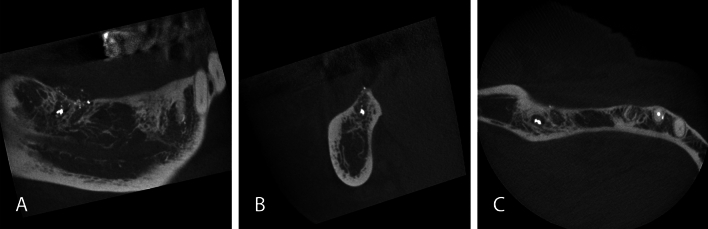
CBCT scan of a 71-year-old female patient with a small FOV (6 × 4 cm) for implant pre-treatment examination in free-end posterior right lower jaw. In the lower right wisdom tooth position a radiopaque concrement of dental filling material with circumferential radiolucency is visible. Furthermore, multiple small radiopaque concrements are recognizable in the residual socket and adjacent to the bone in the soft tissue (fragmented dental material in bone/in soft tissues). In the axial plane dish-like root remnants are identifiable in second premolar site, where implant placement was planned. Sagittal (A), coronal (B) and axial (C) images
